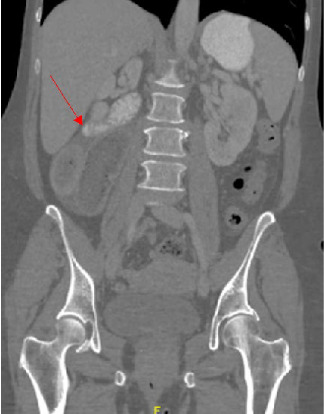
CT showing rightward sweeping duodenum (arrow) leading to jejunum without crossing the midline.
